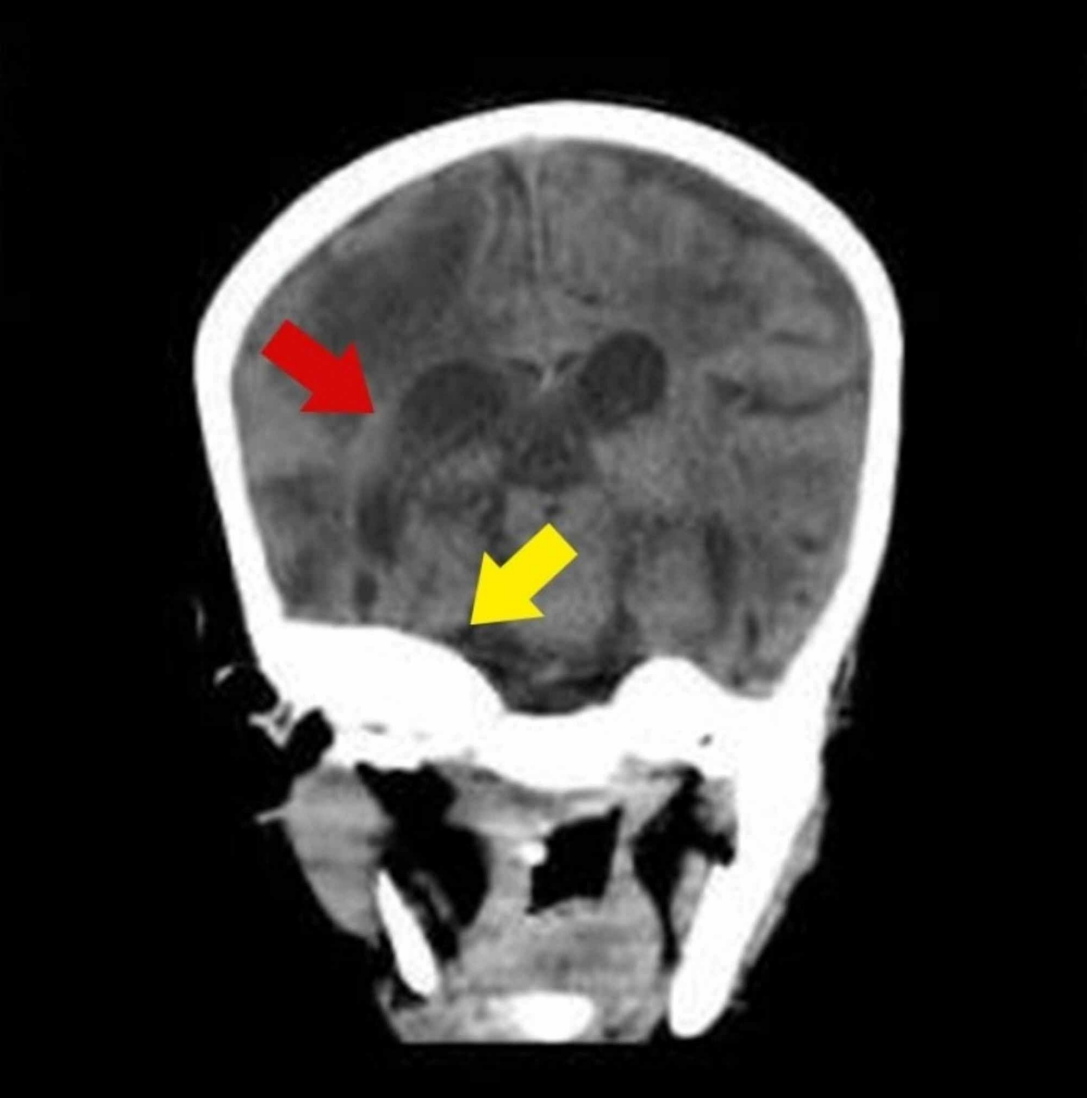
CT scan brain showing right frontal lobe lesion causing mass effect, inflammation, loss of gyri and sulci (red arrow), and cortical thickening of the skull (yellow arrow).
